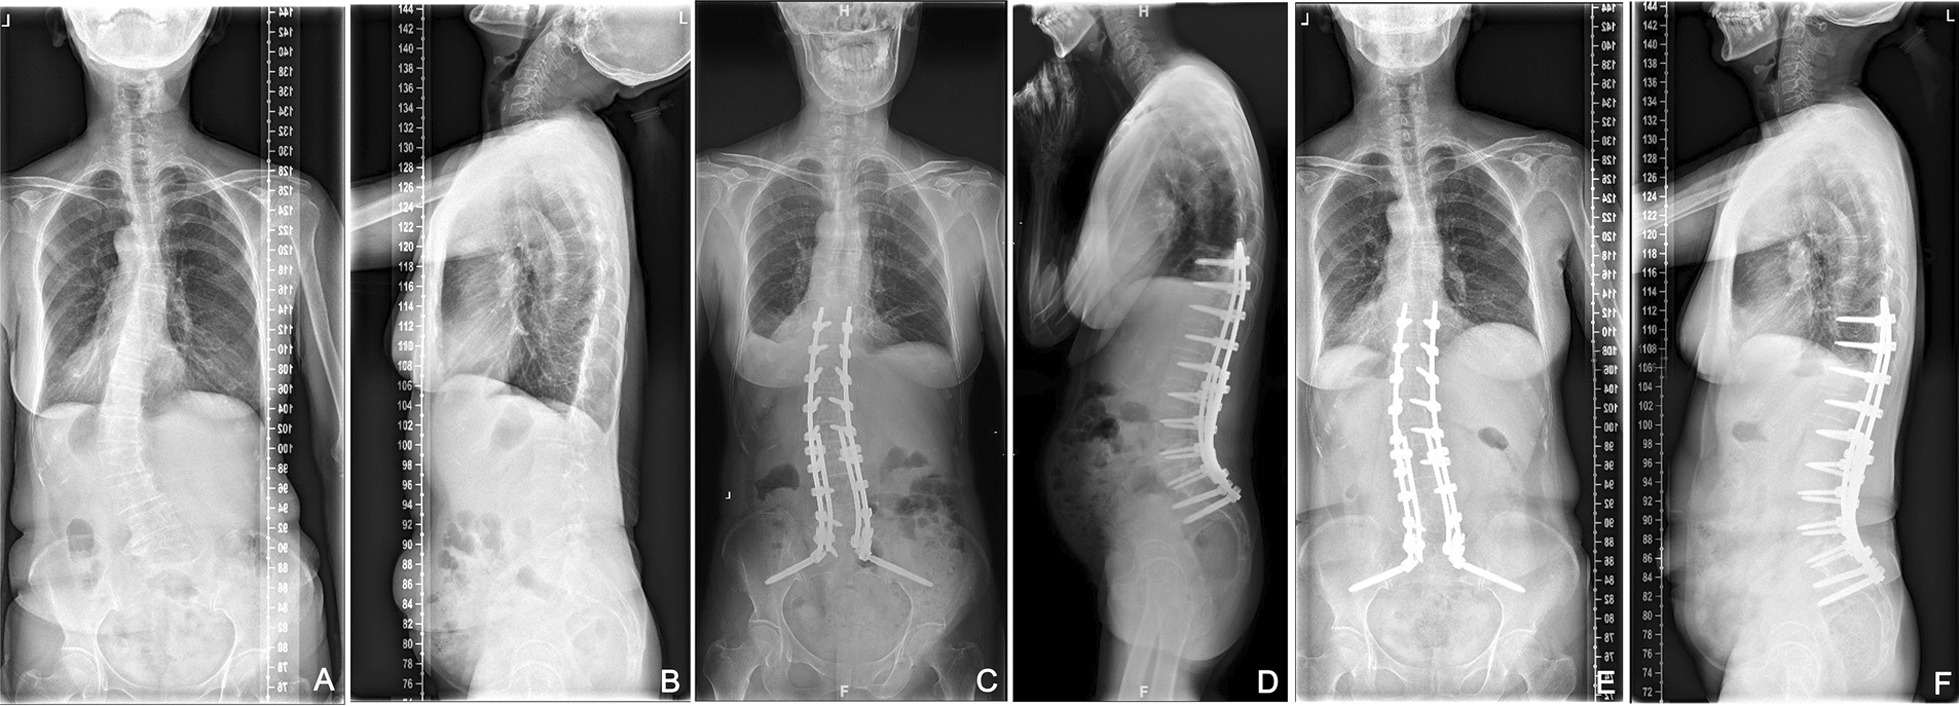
The imaging data of a typical case of degenerative scoliosis before operation and during the follow-up period. The patient is a 65-year-old female with clinical manifestations of low back pain and intermittent claudication. A, B The anteroposterior and lateral X-rays of the whole spine before operation. C, D The anteroposterior and lateral X-rays of the whole spine immediately after operation. E, F The anteroposterior and lateral X-rays of the whole spine after 5 years of follow-up. Comparing the imaging data, it could be found that Ponte osteotomy combined with PLIF technology can effectively reconstruct lumbar lordosis and restore coronal and sagittal plane balance in patients with degenerative scoliosis, and the effects of correction can be maintained for a long time after surgery
